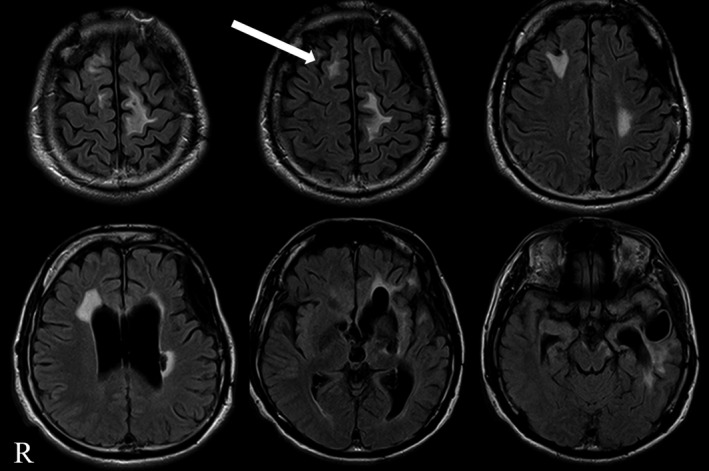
Brain MRI (1.5 Tesla, Fluid‐Attenuated Inversion Recovery; FLAIR). Brain MRI shows multiple old brain contusions, mainly in the left hemisphere. Additionally, several high‐intensity areas are observed, including an area in the right pre‐supplementary motor region, which is indicated by the white arrow
